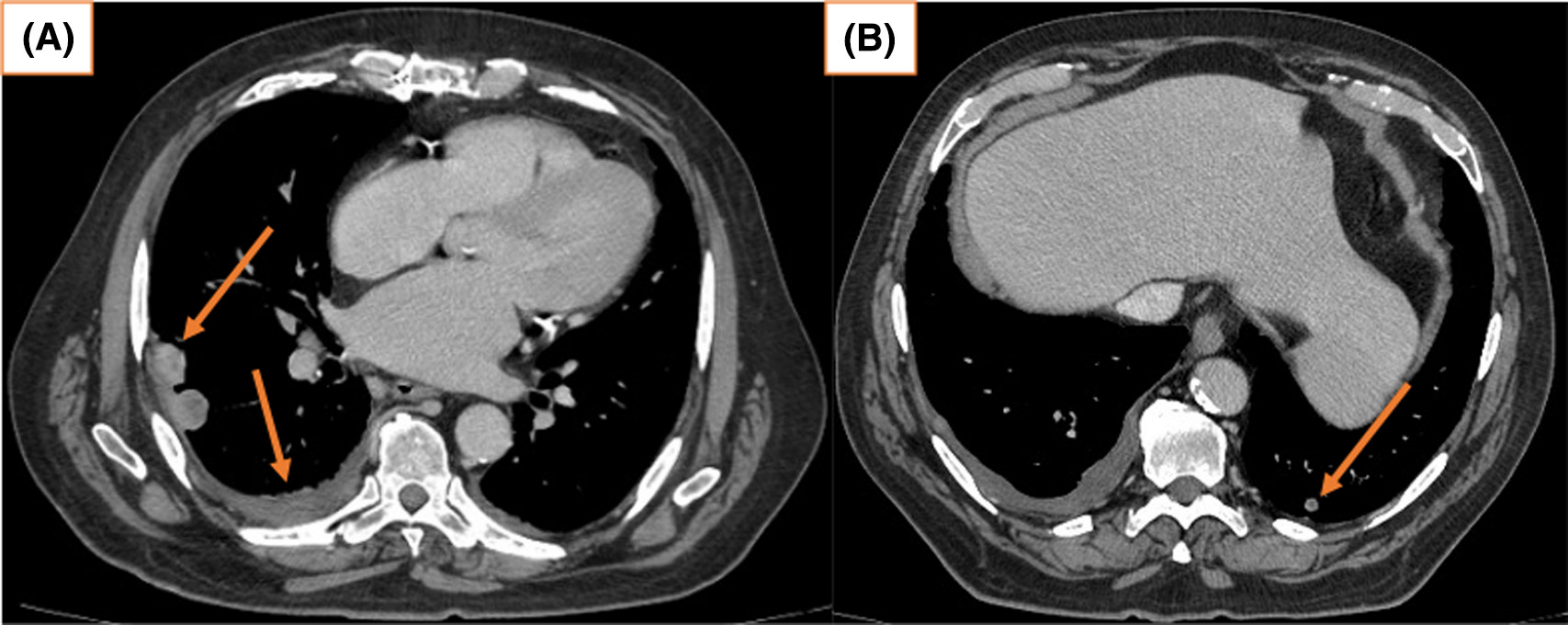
(A, B) October 2022, chest CT after 3 months of treatment showing partial treatment response with improvement in effusion without further thoracocentesis since discharge in July, and a reduction in the size of right pleural and left lung metastases.
